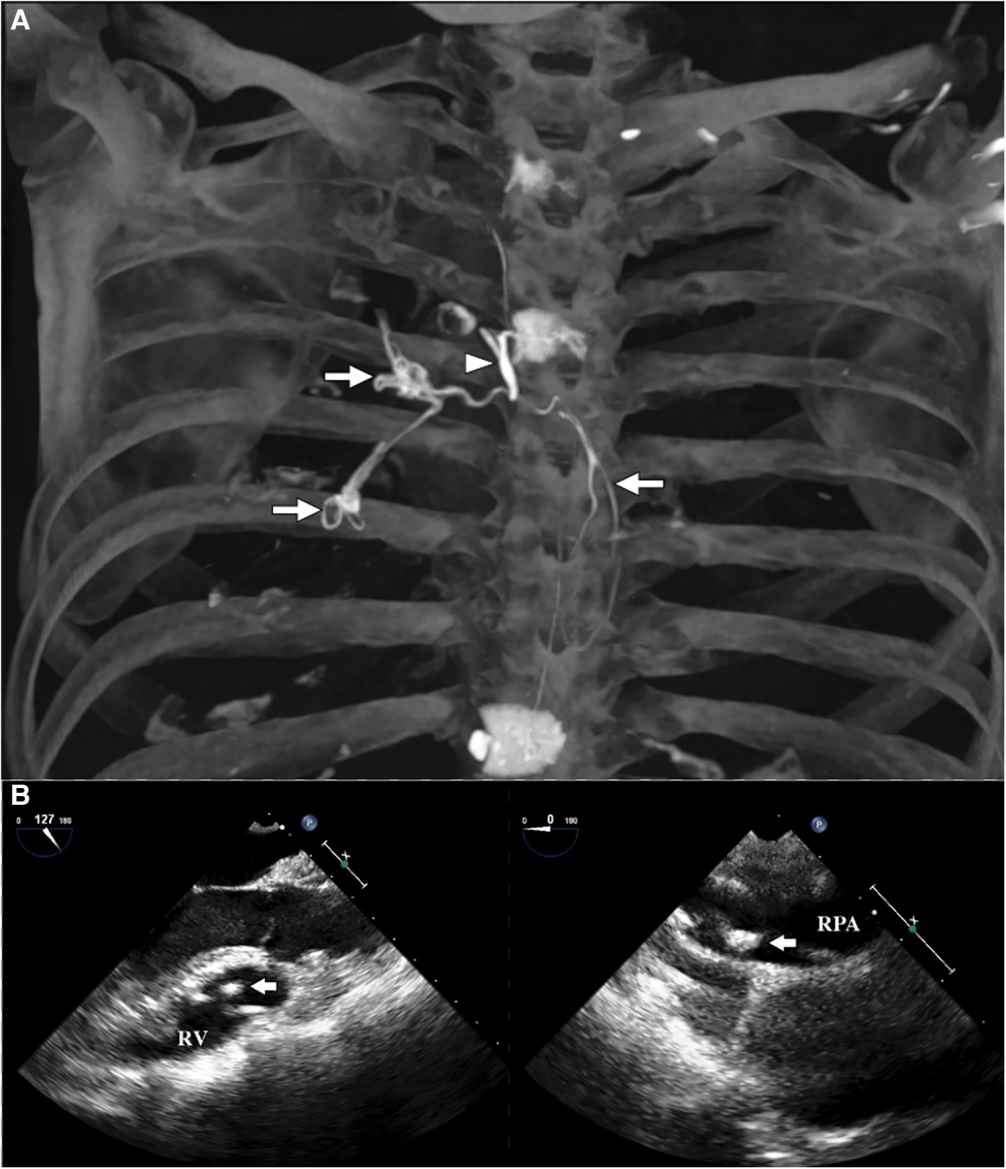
(A) The three-dimensional CT reconstruction demonstrated a tree-branch-shaped high-density foreign material in the RV (long arrow), extending through the MPA and RPA into the ascending branch and middle-lobe branch of the RPA (long arrow), indicating the diagnosis of PMMA cement embolism. The cement seemed to enter the vein system through the azygos vein (short arrow). (B) The transesophageal echocardiography demonstrated a rod-shaped hyperechoic foreign body in the RV and RPA (arrow).
